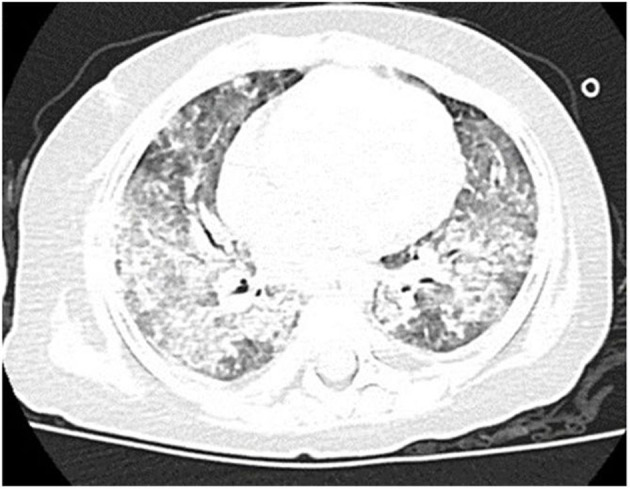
Clinical characteristics of our patient. Chest CT showed disclosed extensive progressive interstitial changes in both lungs on August 2016.
